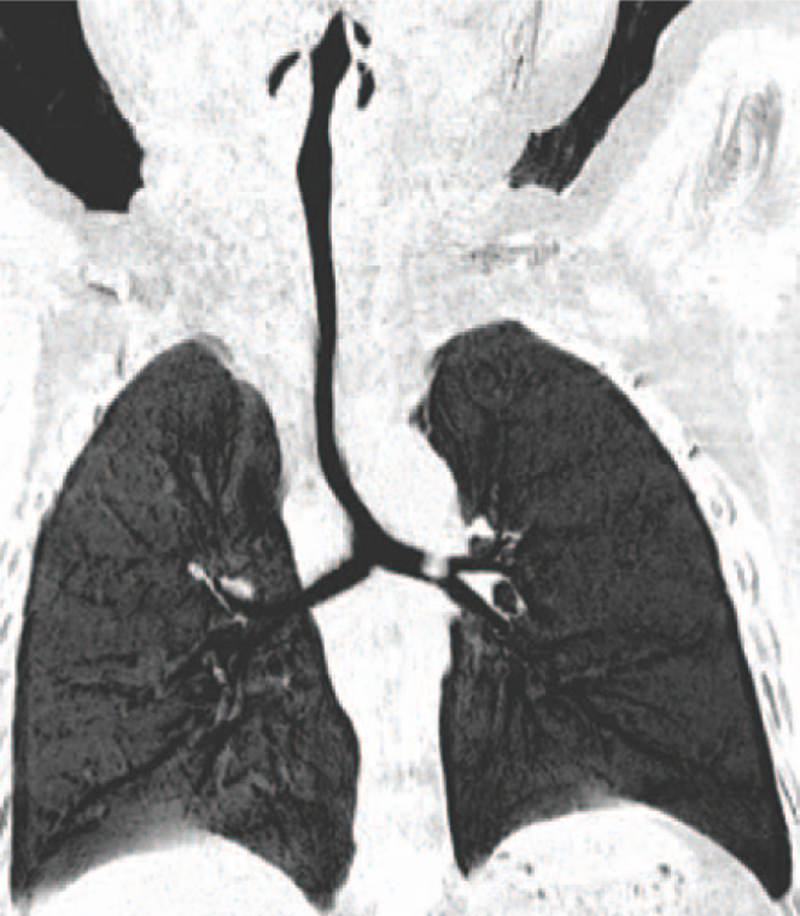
Three-dimensional CT image showing near total occlusion of the left main bronchus and left lung emphysema, a mild degree of tracheal stenosis, and abnormally low tracheal bifurcation at T6 level.
